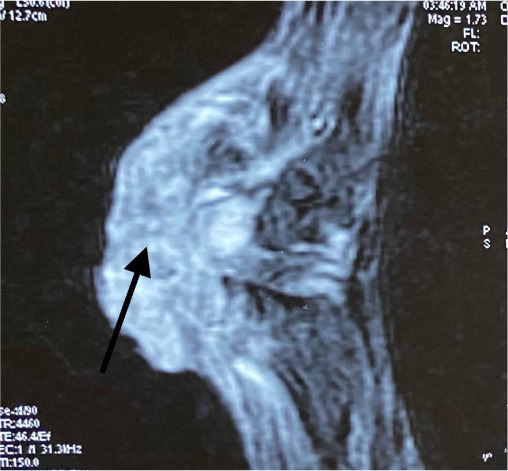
MRI images of the right wrist- (STIR sagittal) showing an ill-defined soft tissue lesion involving the flexor aspect of the distal third of the forearm and wrist (thin black arrow). Lesion involving the pisiform bone (thick black arrow).
